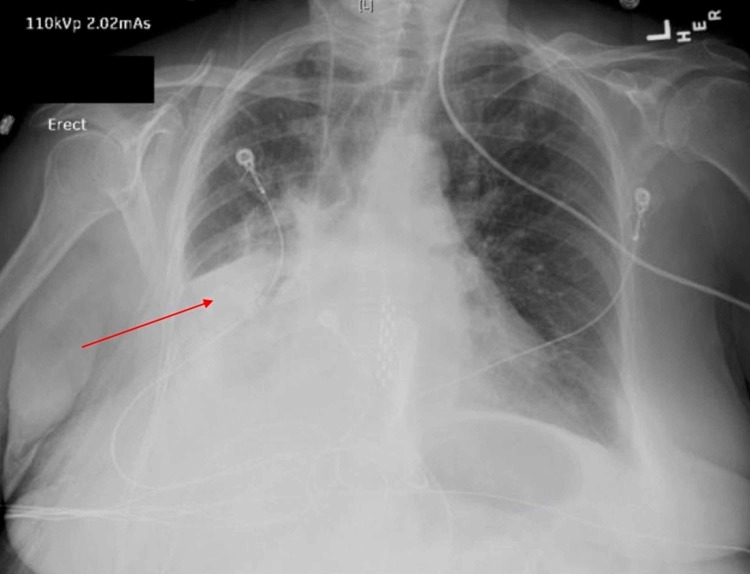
Chest radiograph revealed a large right-sided pleural effusion (red arrow)
